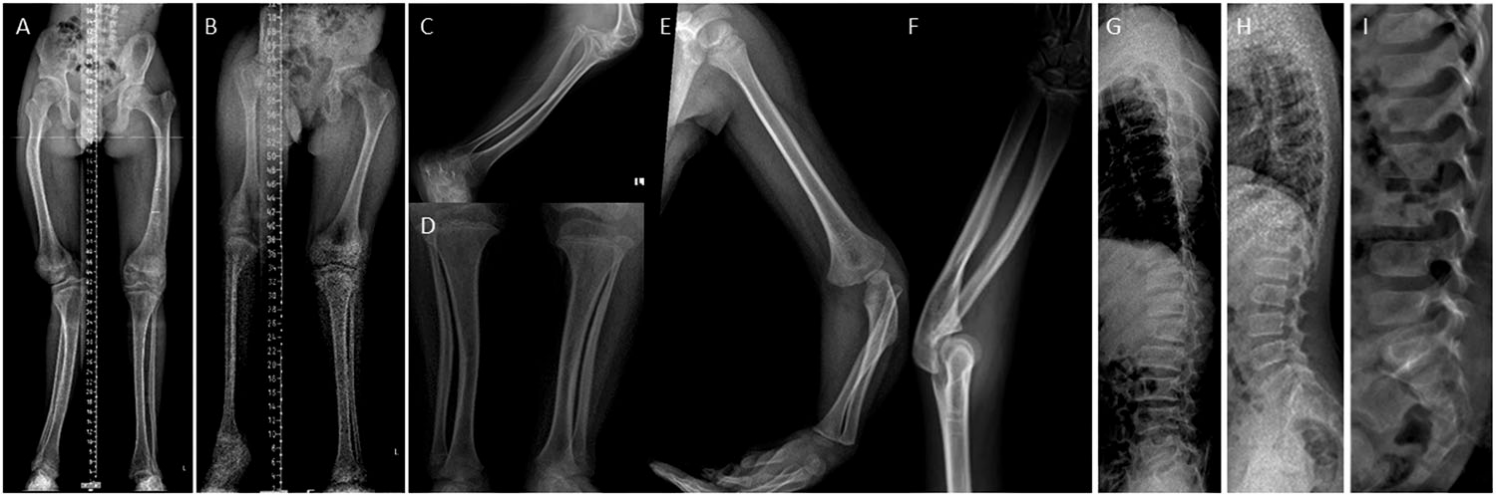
Bowing of long bones in the affected individuals. Radiographs of limbs of probands: proband 1 at 15 years (A), proband 2 at 11 years (B) and proband 3 at 3 years of age (C–F) show bowing of long bones. Bowing of femur (A and B), bowing of the tibia and fibula (A, C, D) can be observed. Irregular epiphyses and metaphyses along with flaring of metaphyses at the knee joint can be noted (D). Variable platyspondyly can be observed in all (G–I).
